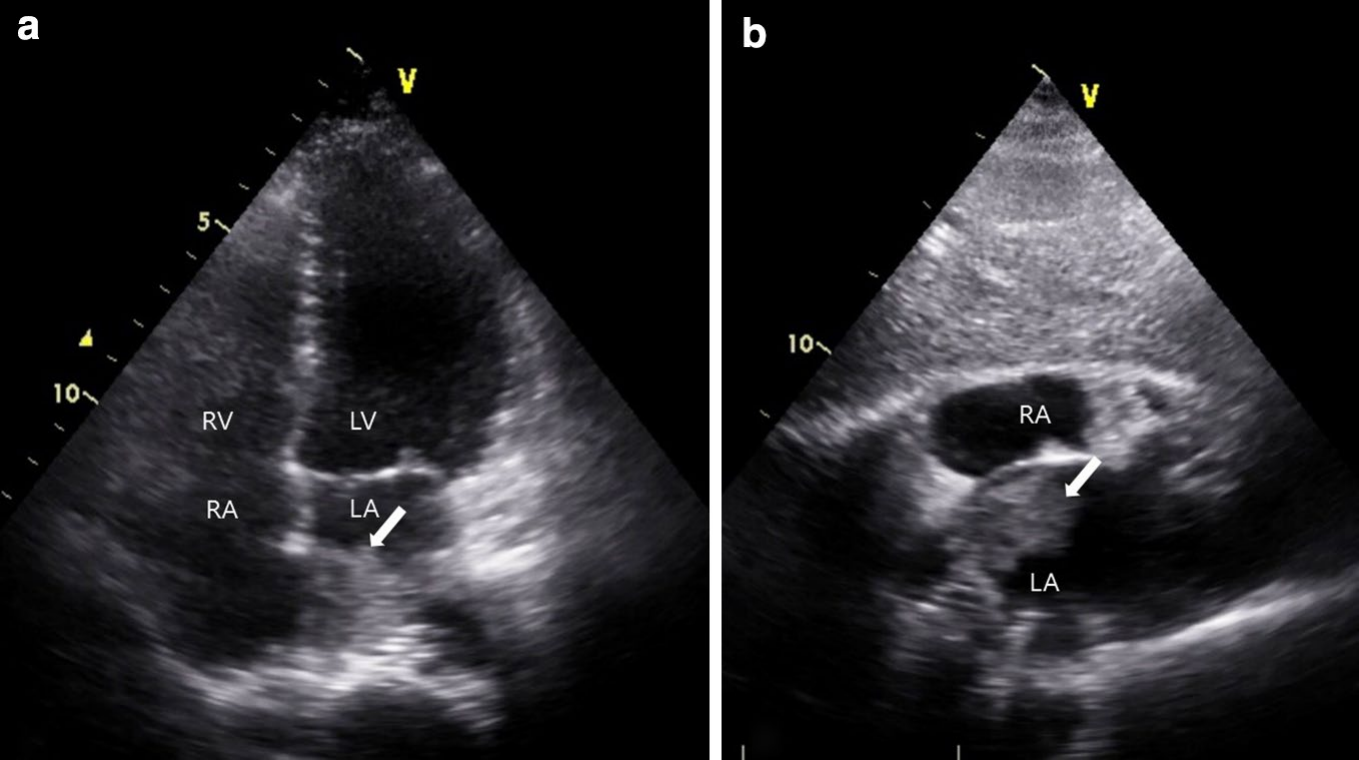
Transthoracic echocardiographic findings. a Mass with irregular margin in the left atrium and unclear site of attachment (in *four chamber view*), b (in *subcostal view*). *LA* left atrium, *RA* right atrium, *LV* left ventricle, *RV* right ventricle
